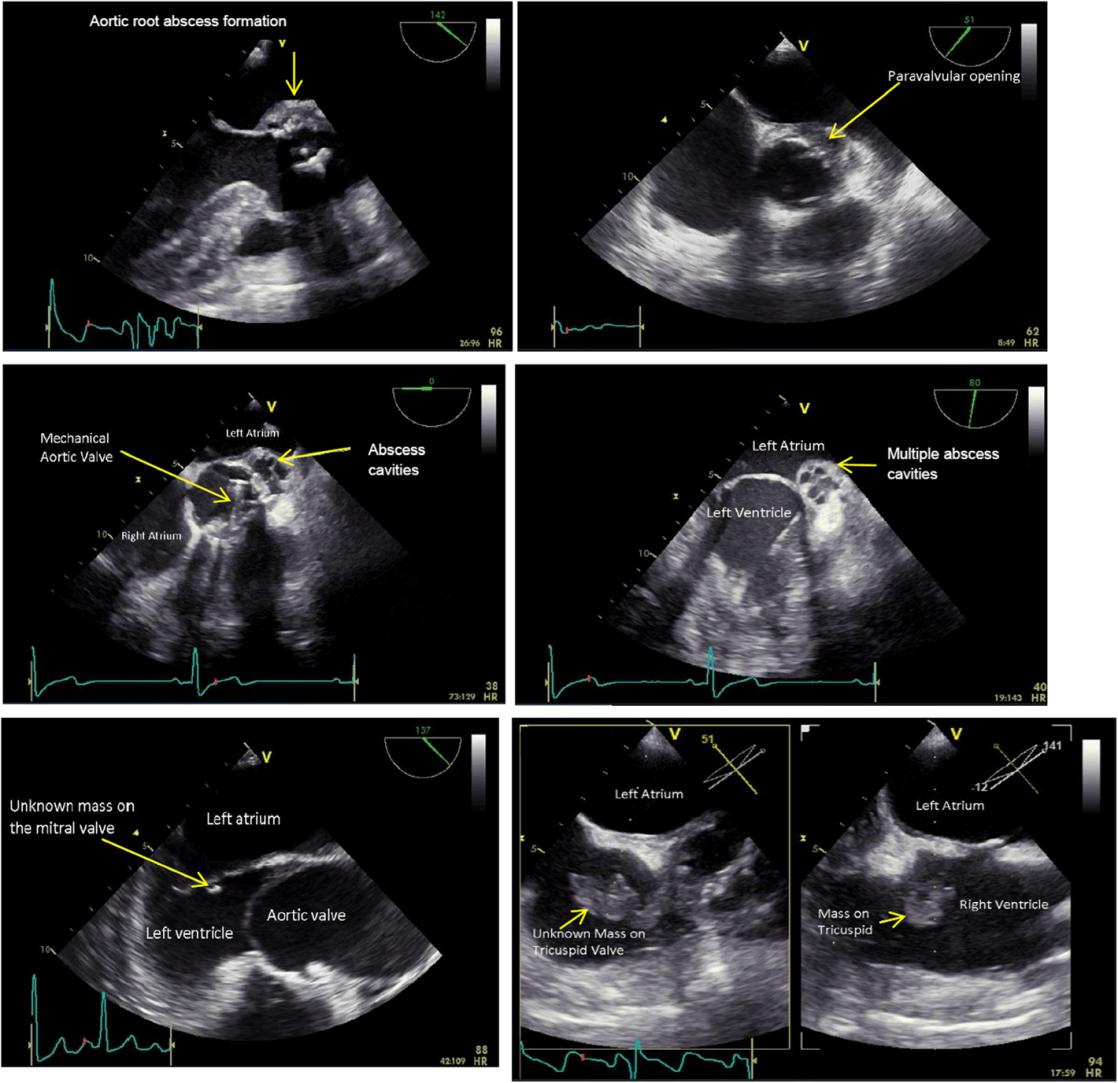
Aortic valve endocarditis with paravalvular abscess formation, transesophageal echocardiographic view
